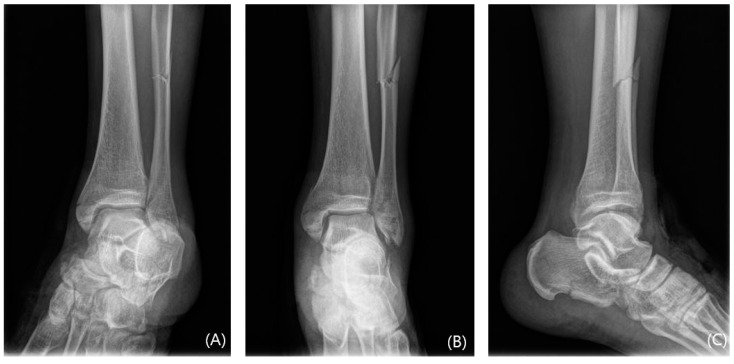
The preoperative mortise, anteroposterior, and lateral radiographs (A–C) of a 47-year-old female with Lauge-Hansen class PER IV ankle fracture. The AITFL fibular avulsion fracture is not visible on these plain radiographs.
